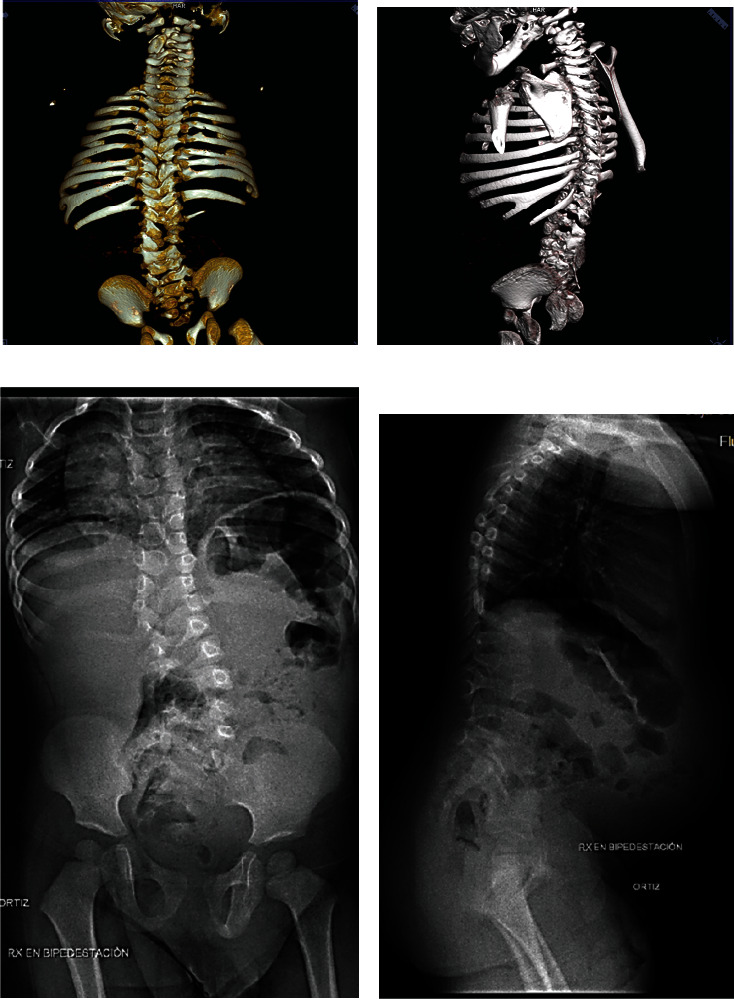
Computed tomography and X-ray imaging of the SCD3 proband. (a) Posterior CT image at three months. Note the disorganized vertebrae in the cervical, lumbar, and sacral spine and the right thoracic hemivertebrae. There are 10 left and 9 right asymmetrically set ribs with posterior proximal fusion and posterior distal fission. (b) 45-degree clockwise posterior CT image at three months. Note posterior rib fusion and fission as well as asymmetrical vertebral shape. (c) Posterior X-ray at one year and eight months. The posterior spine is characterized by the “pebble beach sign,” angulated vertebrae, and scoliosis. (d) Lateral X-ray at one year and eight months. Note the disorganized vertebral bodies in the thoracic and lumbar spine.
